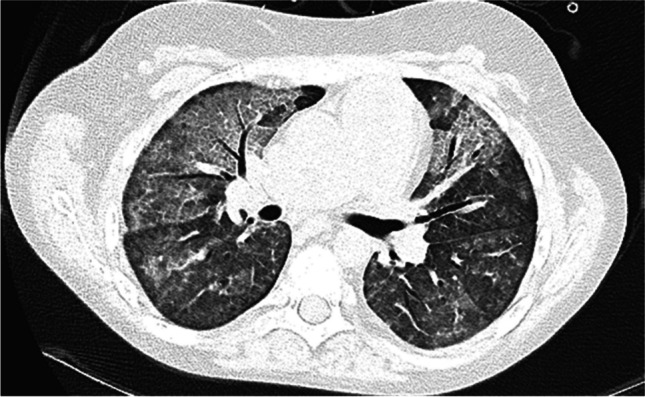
Axial contrast-enhanced lung window computed tomography image in a 12-year-old girl with granulomatosis, polyangiitis, and pulmonary hemorrhage who presented with hemoptysis and anemia. The image shows interlobular septal thickening superimposed on areas of ground-glass opacity, resulting in a characteristic crazy-paving pattern
